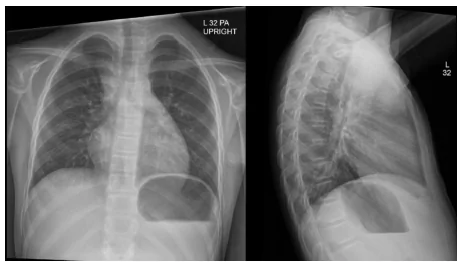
Chest radiograph of a seven-year-old girl with vomiting, diarrhea. and decreased oral intake.
